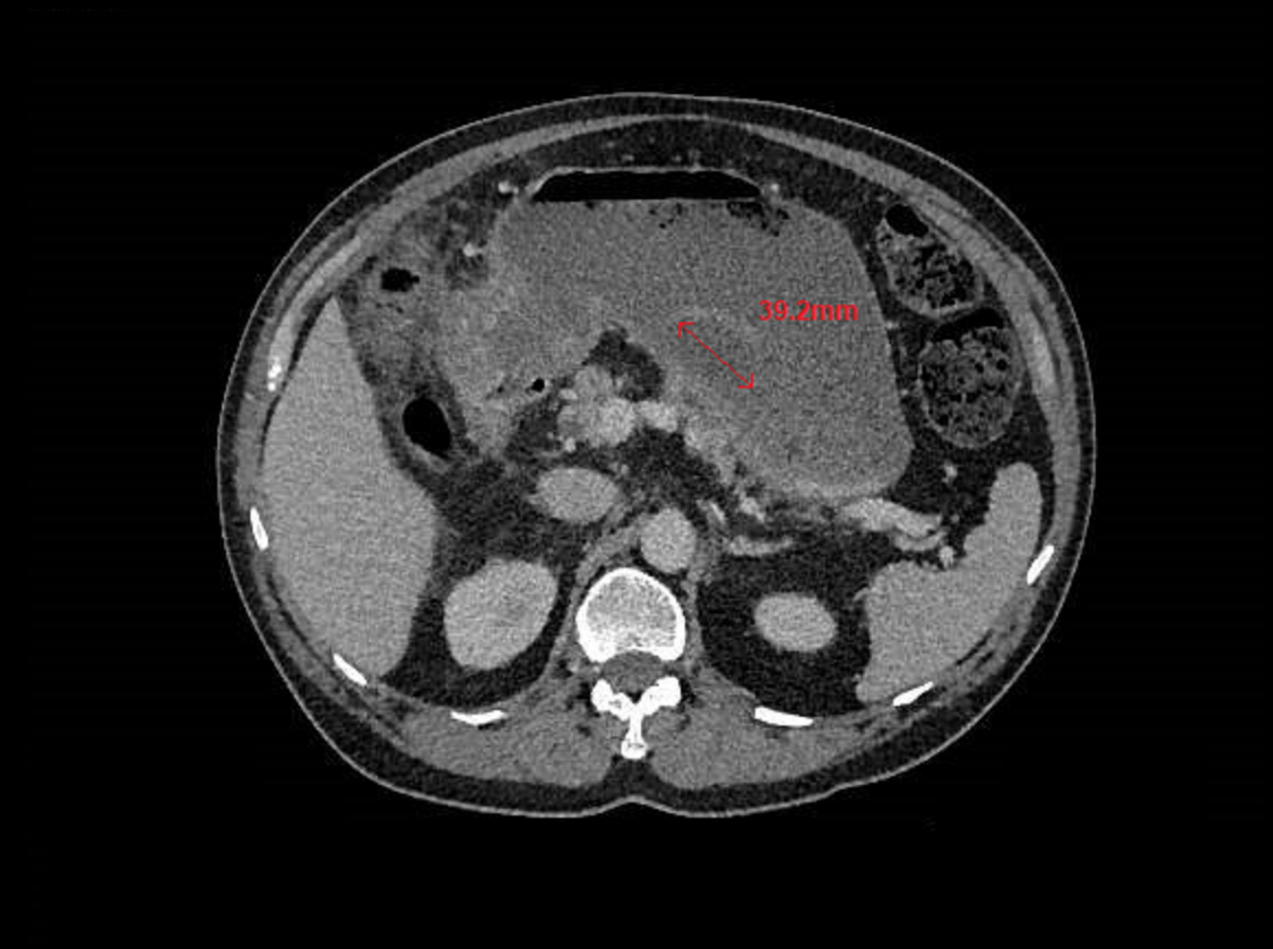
CT demonstrating a 4-cm intraluminal filling defect in the stomach (red arrow)
